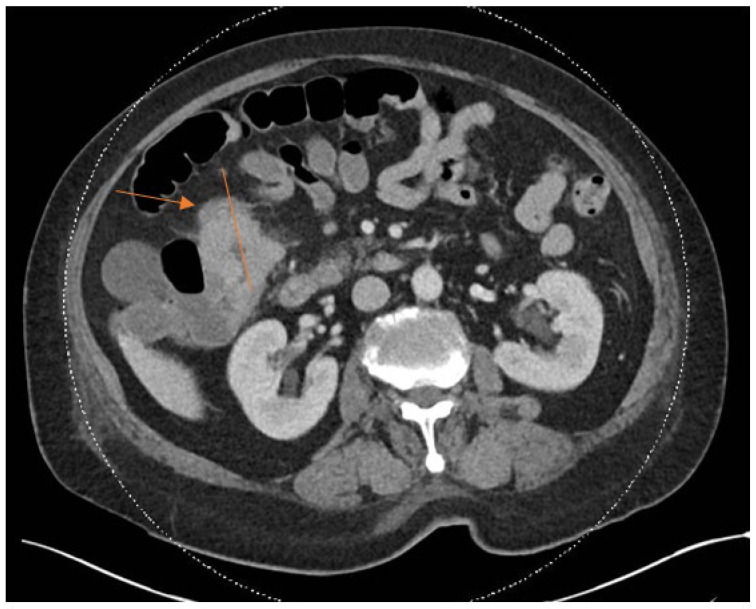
Axial cut of CT-AP scan showing colorectal carcinoma as indicated by arrow.
